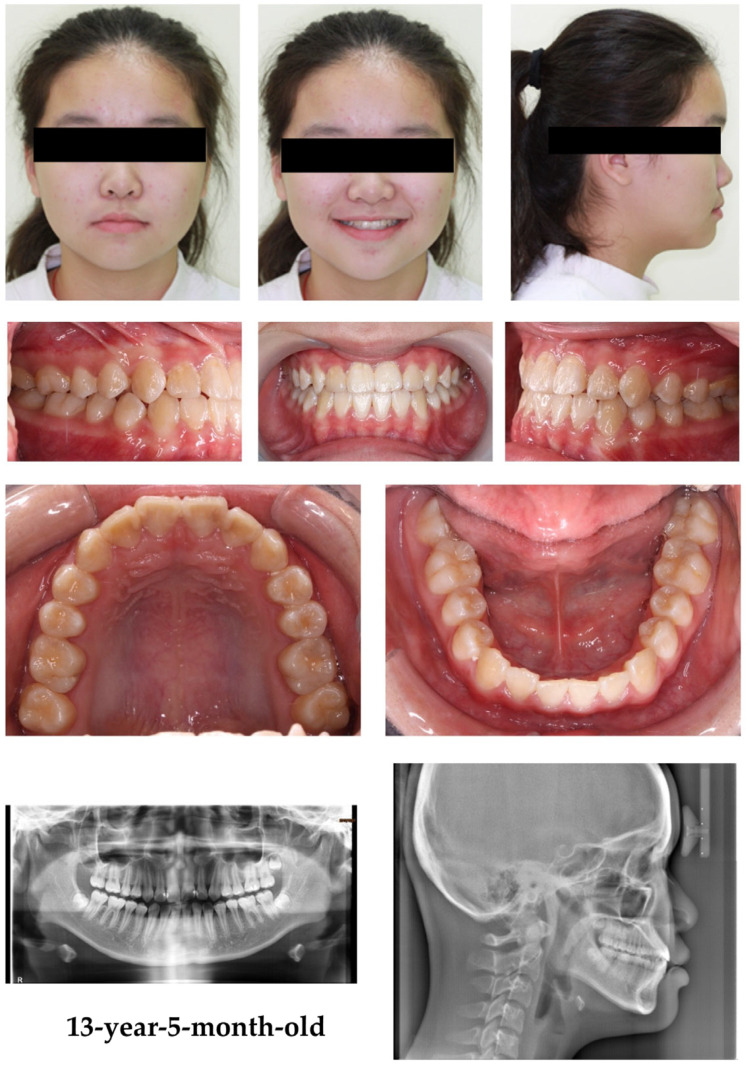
Facial and intraoral photographs, panoramic radiograph, and cephalometric radiograph at 51 months treatment time of a 13-year-5-month-old patient. The facial photographs showed a straight profile, and the intraoral photographs demonstrated acceptable overjet and overbite. The cephalometric radiograph indicated cervical vertebral maturation stage 4.
